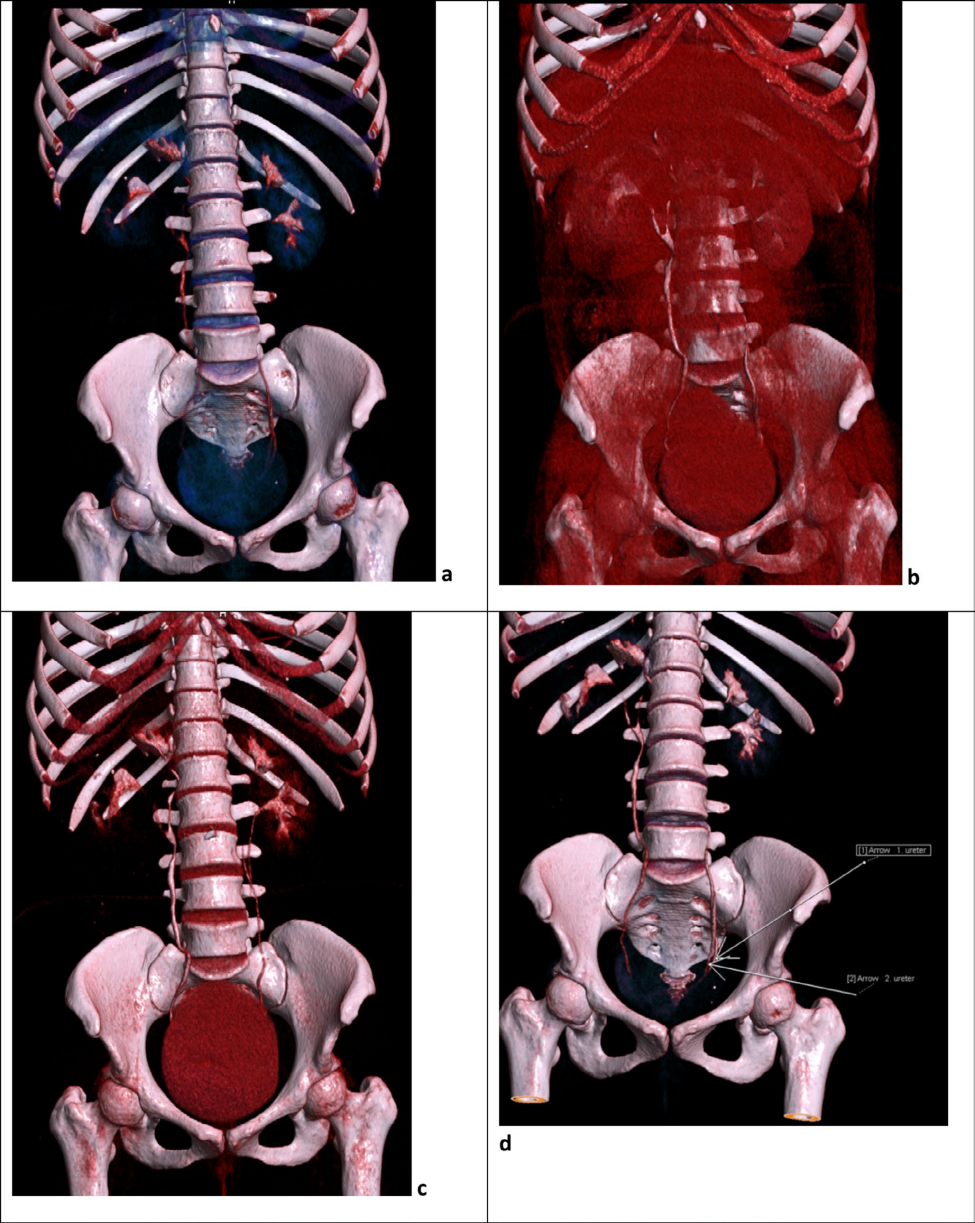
CT volumetric images of the abdomen showing the right duplex collecting system draining into a single ureter and a duplex collecting system with a double ureter (complete duplication with two ureters that drain separately into the bladder) in the left kidney.
